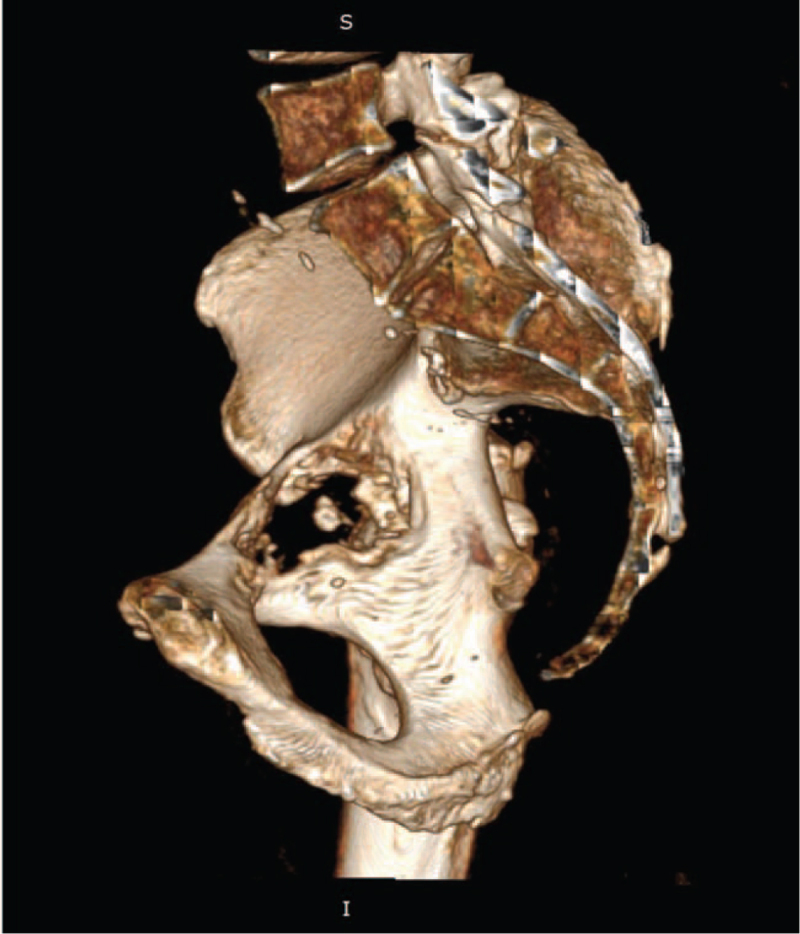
Computed tomography of the right hip joint in 3D imaging, view from the side of the smaller pelvis. Extensive bone loss in the bottom of the acetabulum and quadrilateral plate, after 2.5 yrs of bone remodelling, corresponding to grade IIIA on the Paprosky scale.^[4]^
